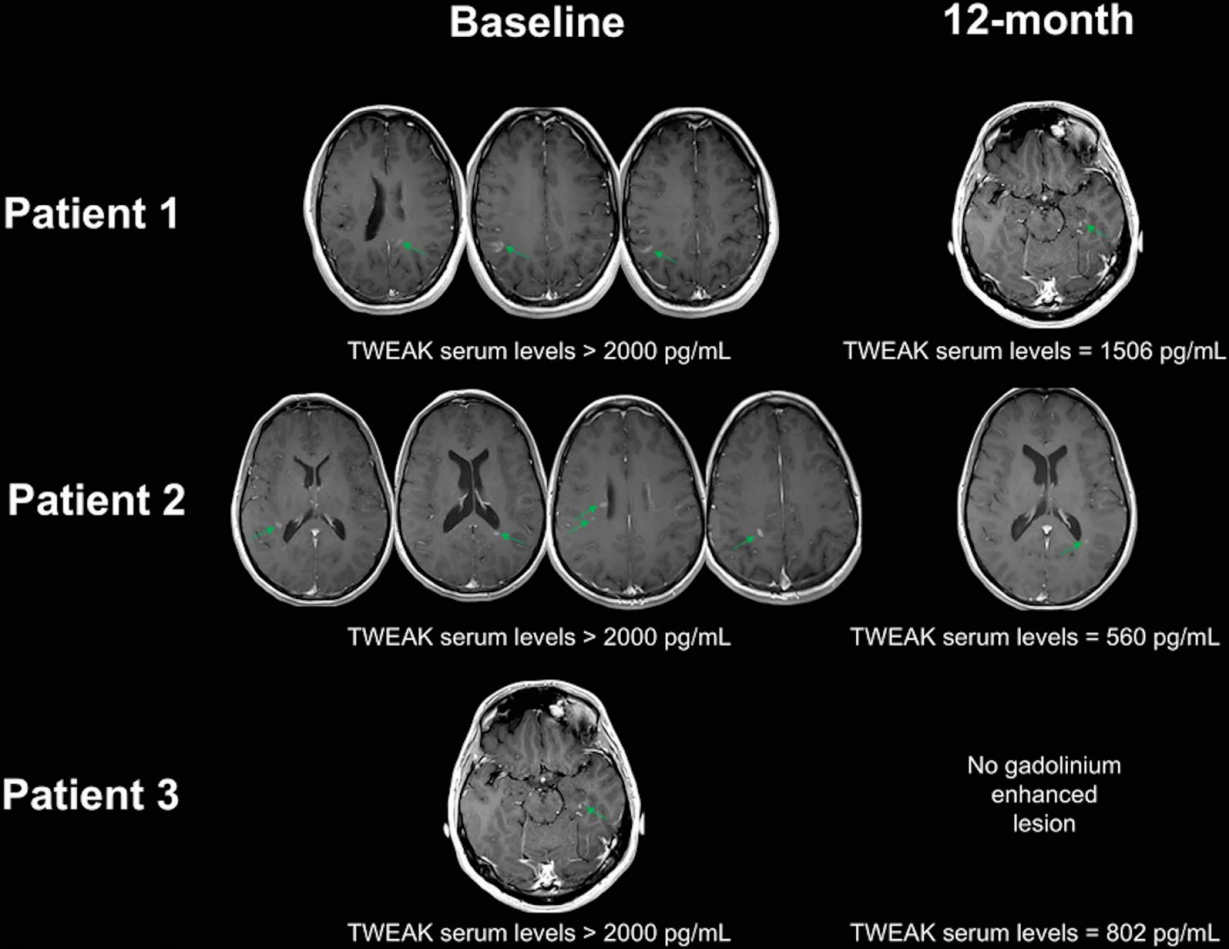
TWEAK serum levels according to gadolinium enhancement. For 3 patients, baseline TWEAK serum levels were particularly elevated and were superior to the highest standard of the TWEAK ELISA calibration curve (> 2000 pg/mL). The brain MRI (T1 after gadolinium infusion) are presented at baseline and 1 year. TWEAK serum levels at 1 year are also reported
